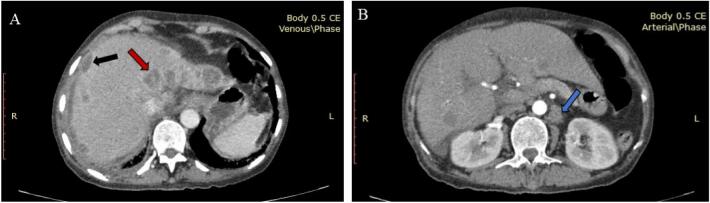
CT scan showing subcapsular deposits-frosted liver (black arrow) and parenchymal deposits (red arrow) (A). Para-aortic lymphadenopathy (blue arrow) (B). (For interpretation of the references to colour in this figure legend, the reader is referred to the web version of this article.)
